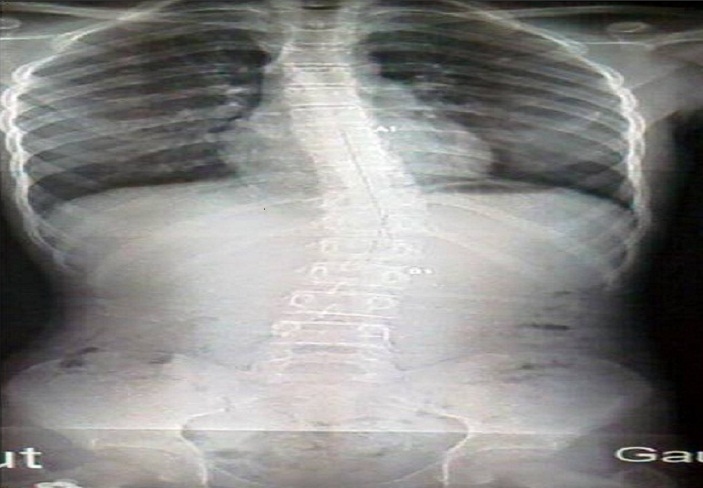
La radiographie standard du rachis de face: objective une scoliose D8-L2 gauche de 25
